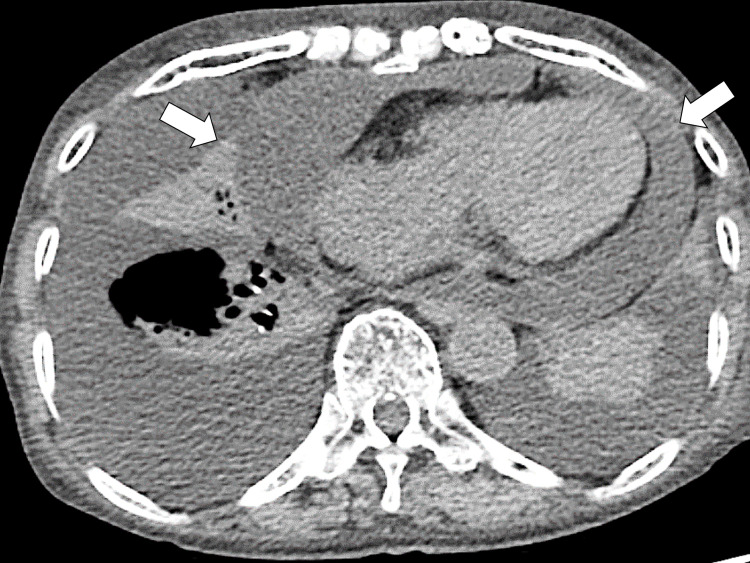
Cardiac tamponade on the subsequent CT (arrows) CT: computed tomography
